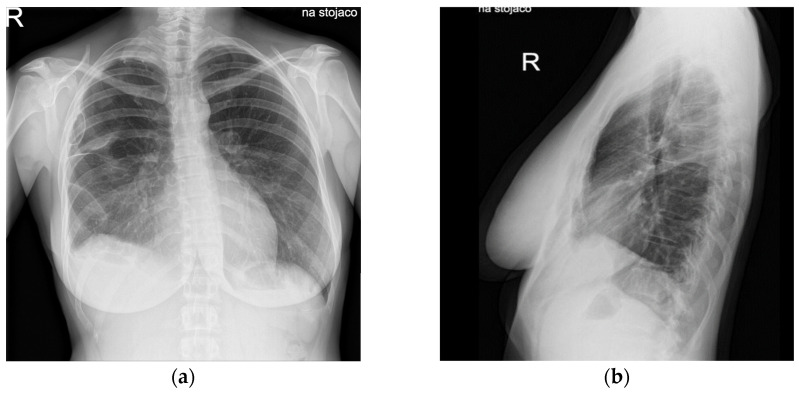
Chest X-ray showing pleural adhesions, fibrotic strands and increased subpleural markings in the right lung in August 2022: (a) PA projection; (b) right-lateral projection *(R—right side marker)*.
